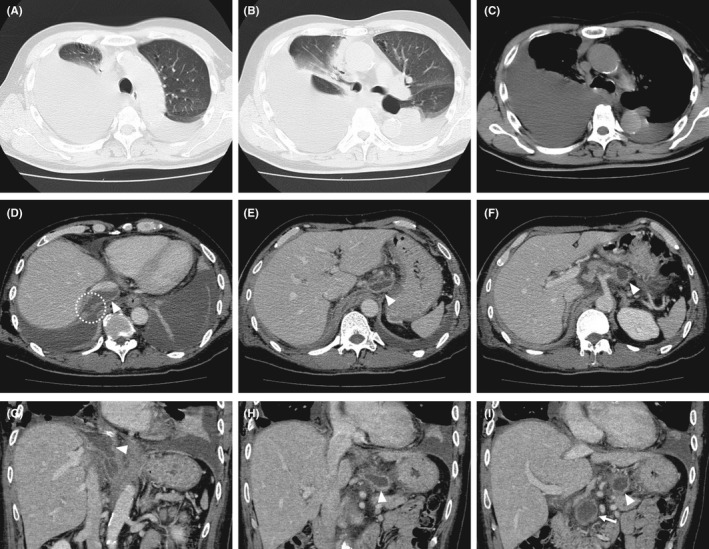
Chest computed tomography (CT) performed at the initial examination (A‐C) demonstrated right side‐predominant bilateral pleural effusion. Axial (D,E) and coronal (G,H) contrast‐enhanced abdominal CT images demonstrated a fluid accumulation in the subphrenic region (arrowhead). A fistula located between the pleural cavity and area of fluid accumulation in the subphrenic region (dotted circle) was suggested (D). Axial (F) and coronal (I) contrast‐enhanced abdominal CT images showed a pseudocyst (arrowhead) in the body of the pancreas that communicated with the fluid accumulation in the subphrenic region. Another pseudocyst (arrow) was apparent in the head of the pancreas (I)
